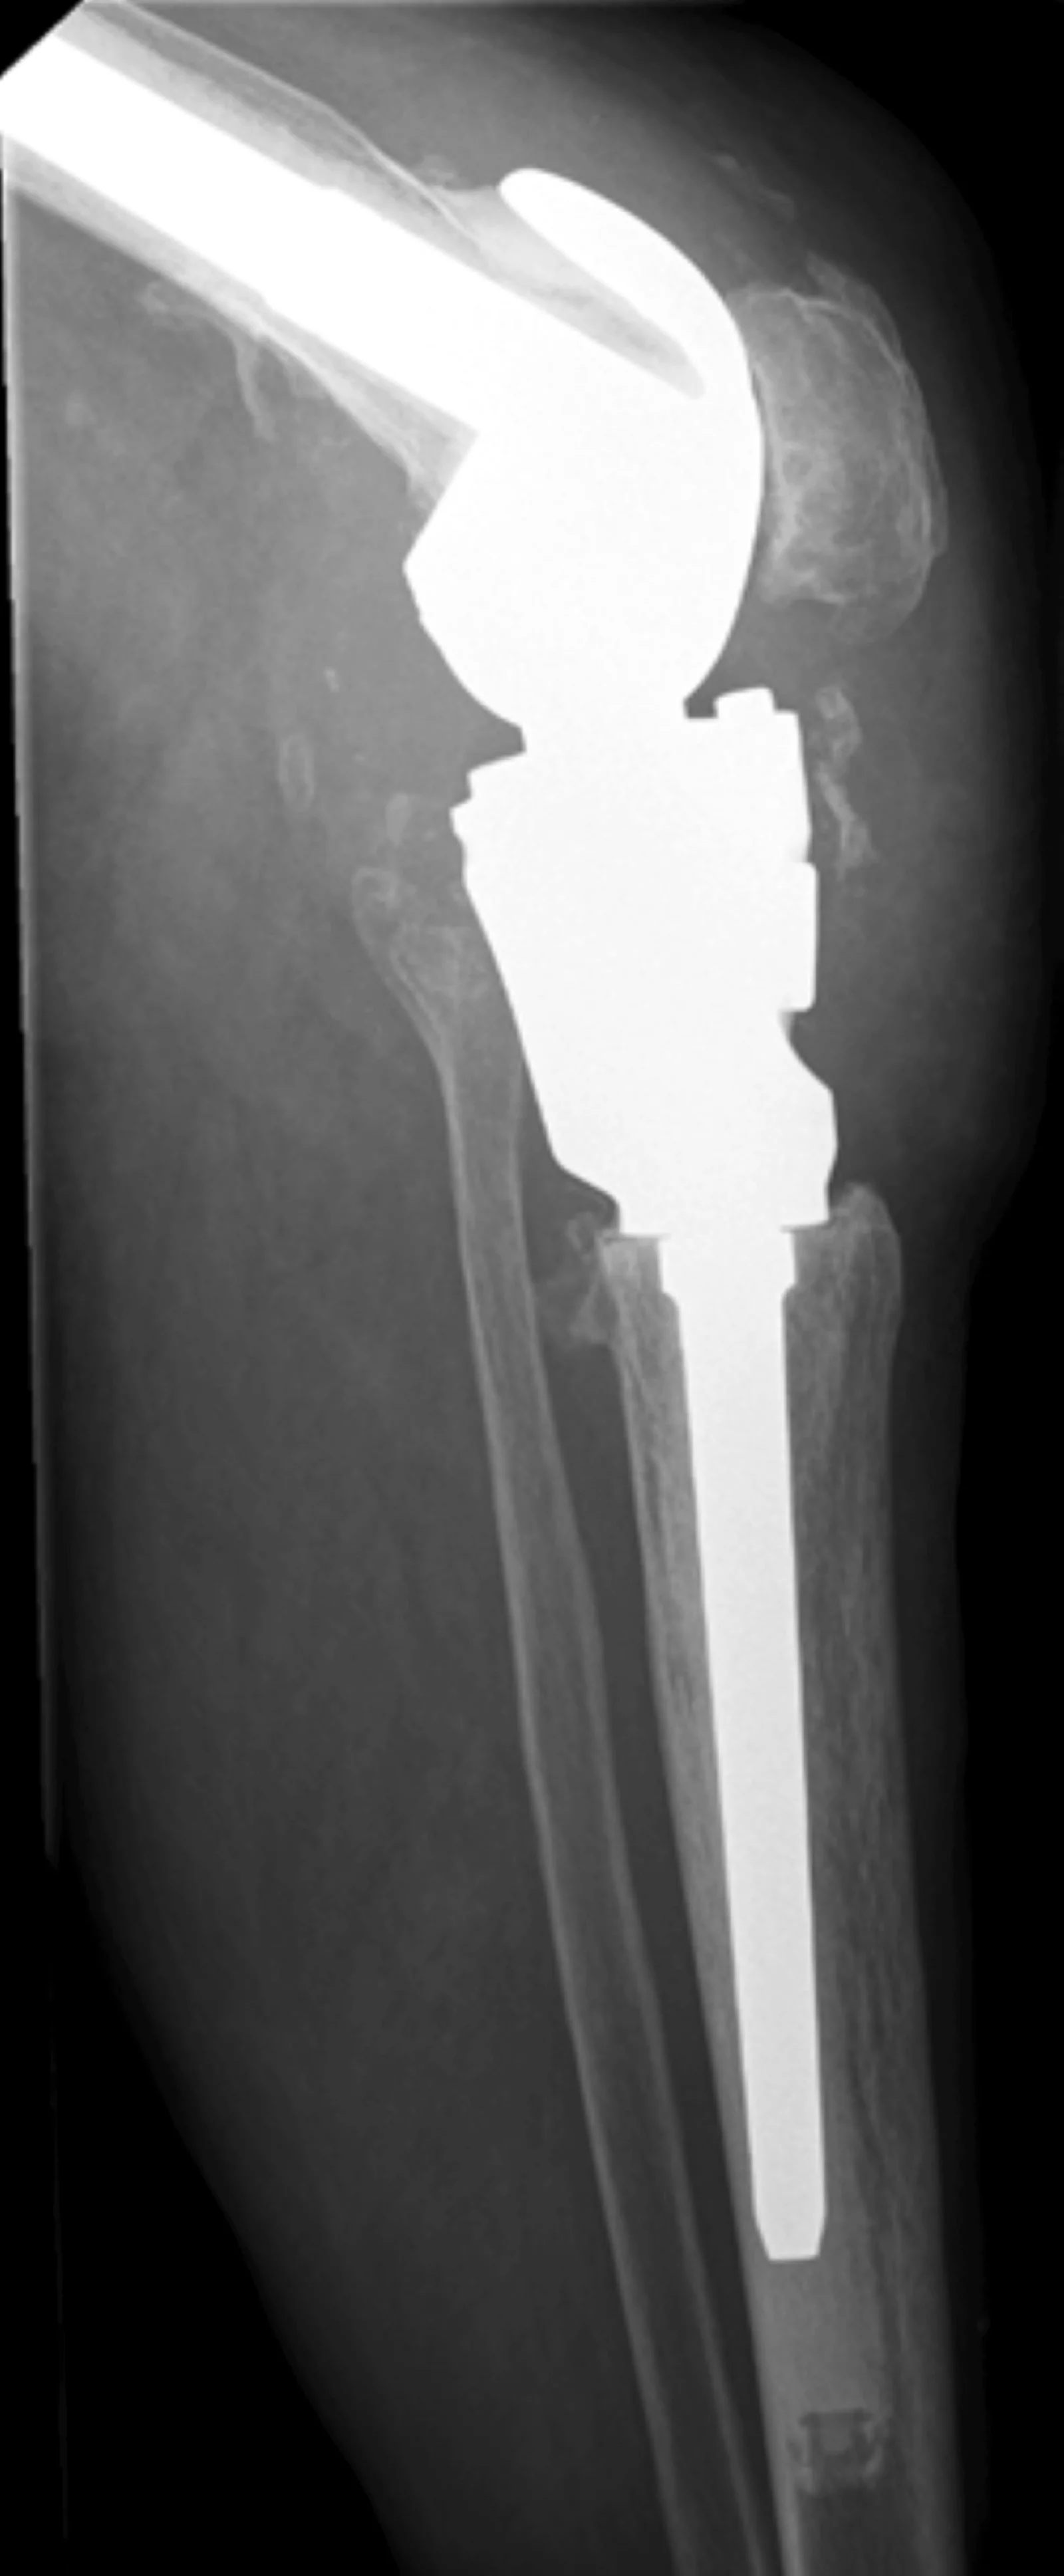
Lateral radiograph of the left knee one year postoperatively showing appropriate implant positioning and no complications after revision arthroplasty.
